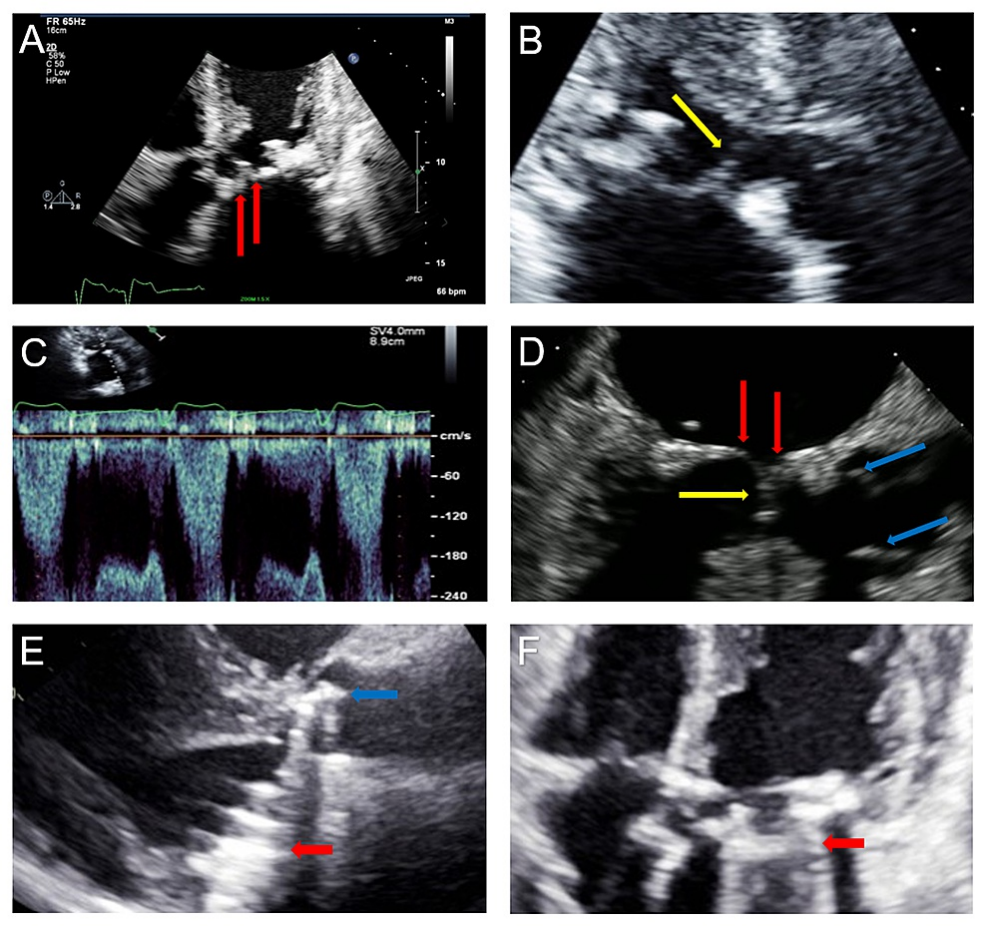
Multimodality echocardiography. (A) Apical five-chamber view of mitral valve bioprosthetic leaflets (red arrows) at start of diastole. (B) Parasternal long-axis view of the mitral valve leaflets in LVOT (yellow arrow) during systole. (C) Pulse wave spectral Doppler at the LVOT showing elevated subvalvular velocity. (D) Pre-operative transesophageal echo. Transducer angle 150° shows the mitral valve (red arrows) during systole, with anterior mitral valve leaflet protruding into the LVOT (yellow arrow), while the aortic valve is open (blue arrows). (E) Two-month post-operative echo (parasternal long-axis view). Red arrow represents the mechanical mitral valve; blue arrow represents the mechanical aortic valve. (F) Two-month post-operative echo (four-chamber view). Red arrow represents the mechanical mitral valve. LVOT: left ventricular outflow tract
